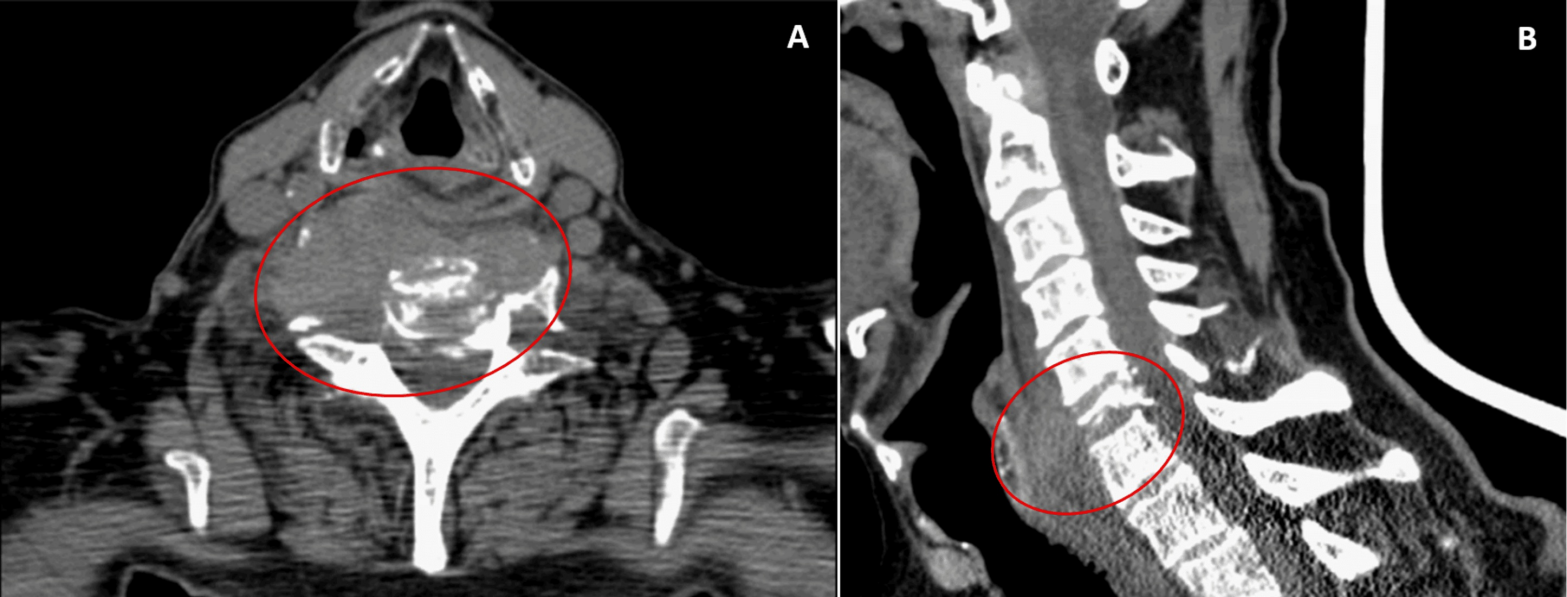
Cervical spine CT scan cervical showing the presence of an infiltrative soft tissue mass in the prevertebral topography of C6, and diffuse hypodensity of the body and right transverse process of the C6 vertebra with extensive lytic areas and cortical lysis (A); subtotal collapse of the vertebral body associated with pathological fracture, with marked reduction in the amplitude of the spinal canal at the C6 level with probable spinal cord compression (B).
